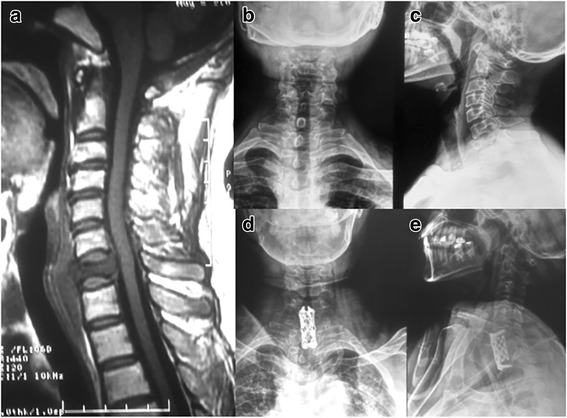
A 47-year-old female with lung cancer was admitted because of severe neck pain and upper extremity numbness. Mid-sagittal T1 MR image (a), anteroposterior (b), and lateral (c) radiographs showing C7 body pathological fracture and encroachment of spinal canal. Anteroposterior (d) and lateral (e) radiographs were obtained after C7 anterior cervical corpectomy and fusion (ACCF). The patient’s symptoms were significantly relieved
